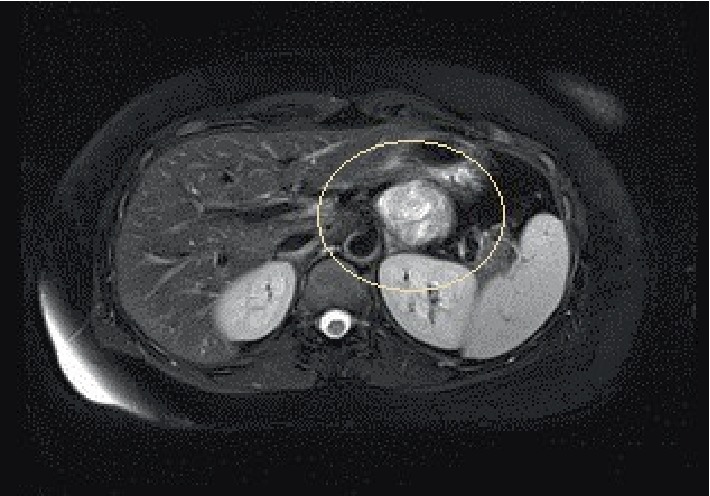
Magnetic resonance imaging (MRI) confirmed a 4.6 cm relatively well circumscribed, hypervascular, enhancing mass (circled) in the distal pancreatic body with no associated pancreatic ductal dilatation.
